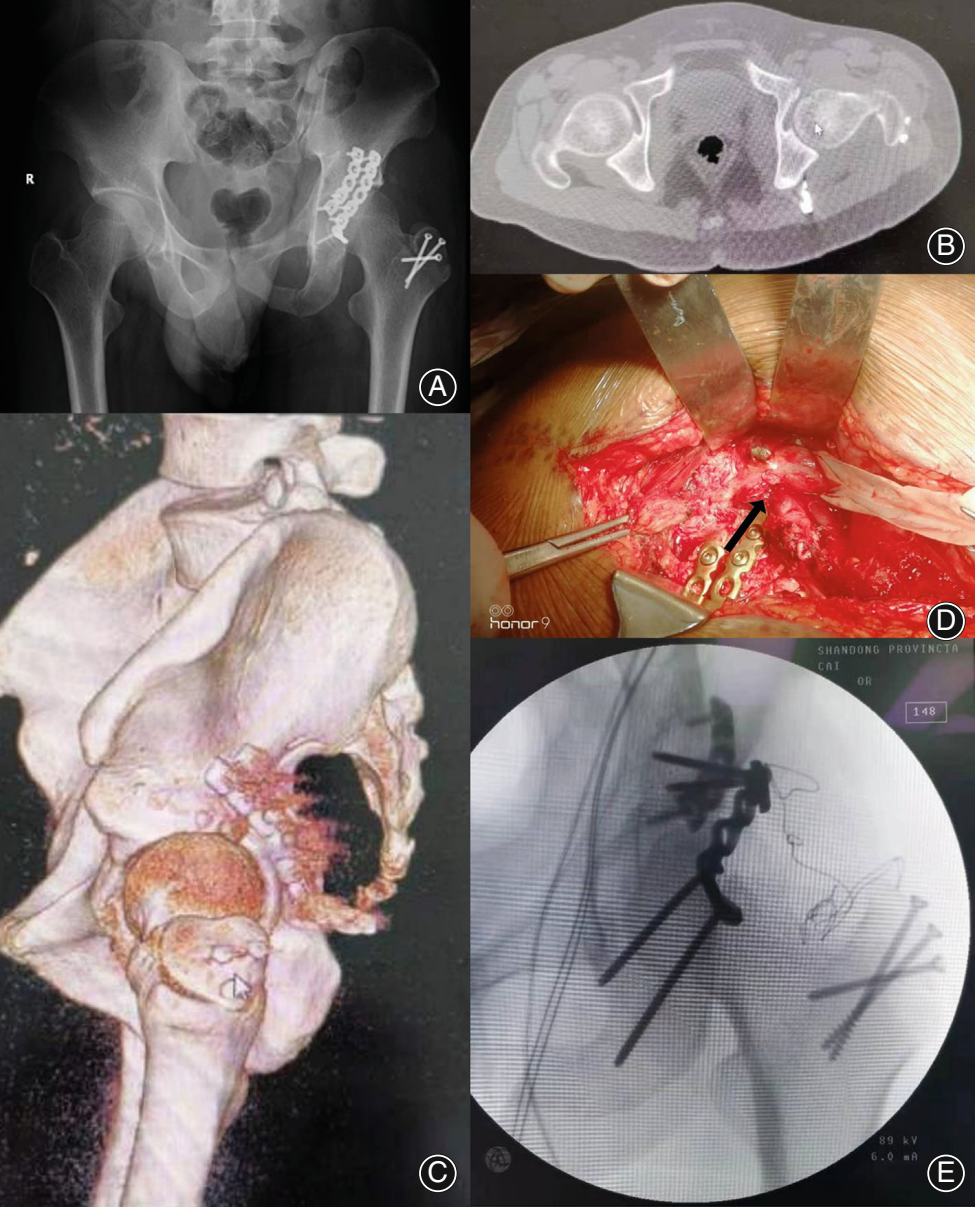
(A) The anteroposterior radiograph displays an implant on the posterior column of the acetabulum with a unique shape and abnormal positioning. (B, C) Computed tomography scans depict the implant situated directly within the region of the sciatic nerve. (D) Intraoperative view showing partial piercing and tight compression of the sciatic nerve under the distal end of the misplaced implant, and the sciatic nerve compression site is adjacent to the two plates and the surrounding soft tissue proliferates (arrows). (E) Postoperative anteroposterior radiograph indicating successful reduction and internal fixation achieved during revision surgery.
